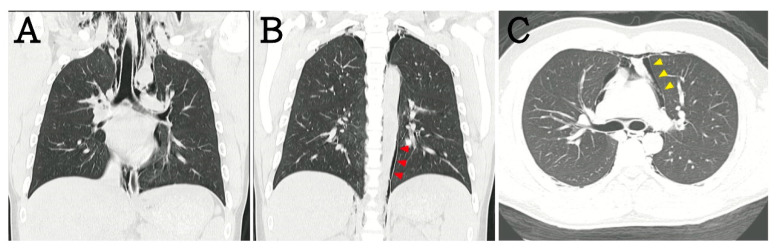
In computed tomography (CT) scans, these results were also noted, including (A) subcutaneous emphysema, (B) periaortic gas, and (C) pericardiac gas.
